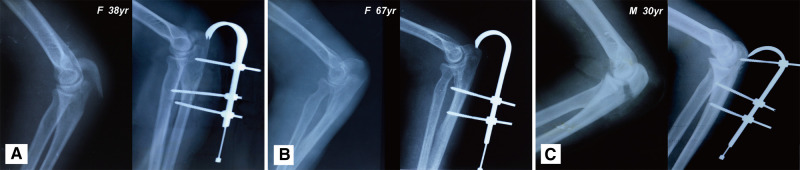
Preoperative and postoperative X-rays of the elbow joint. Typical cases treatment with J-EF in our study. (A) Female patient of 38 years, car accident; (B) female patient of 67 years, fall from height; and (C) male patient of 30 years, sports injury.
